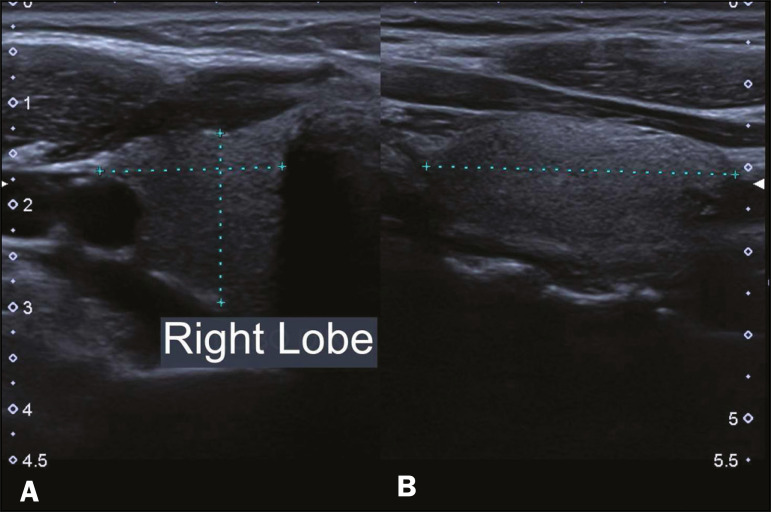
Thyroid ultrasound examination of a euthyroid child. The right thyroid lobe (between the markers) is observed in the transverse section for laterolateral measurement (A) and in the longitudinal section for craniocaudal and anteroposterior measurements (B).
